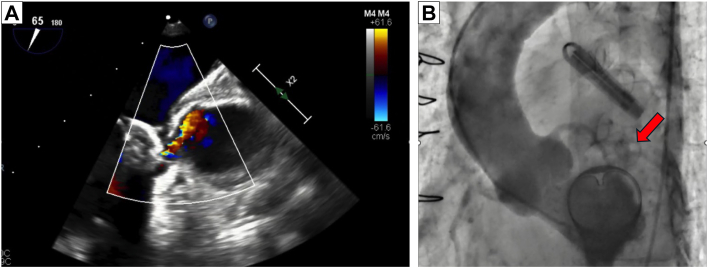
(A) Transesophageal echo image, with Doppler signal detected from the left ventricle to the aneurysm sac. (B) Left ventriculography showed contrast extravasation from the left ventricle (arrow).
